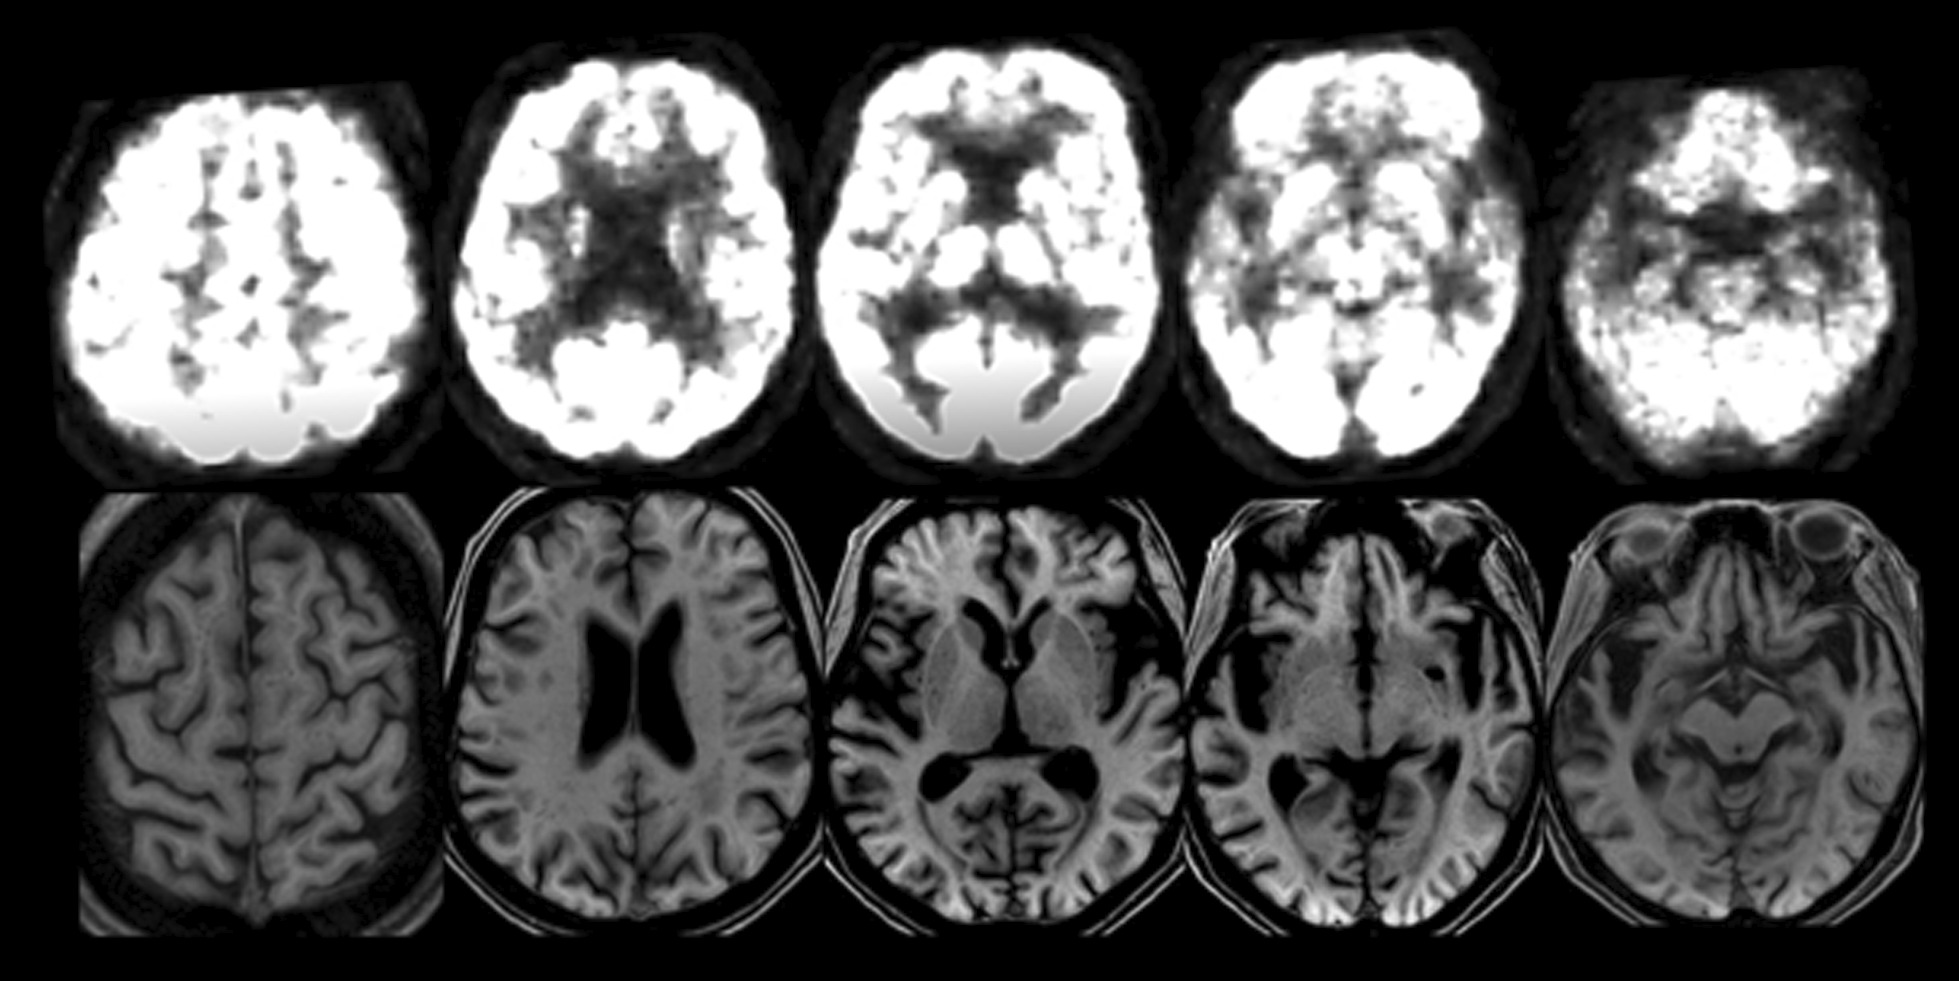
Upper row: ^18^FDG-PET scan showing bilateral hypometabolism in the anterior temporal cortex. The metabolism of the orbitofrontal cortex as well as the caudate nucleus and thalamus is bilaterally normal. Lower row: T1-weighted MRI showing severe bilateral anterior temporal lobe atrophy with relative sparing of the frontal, parietal, and occipital lobes
